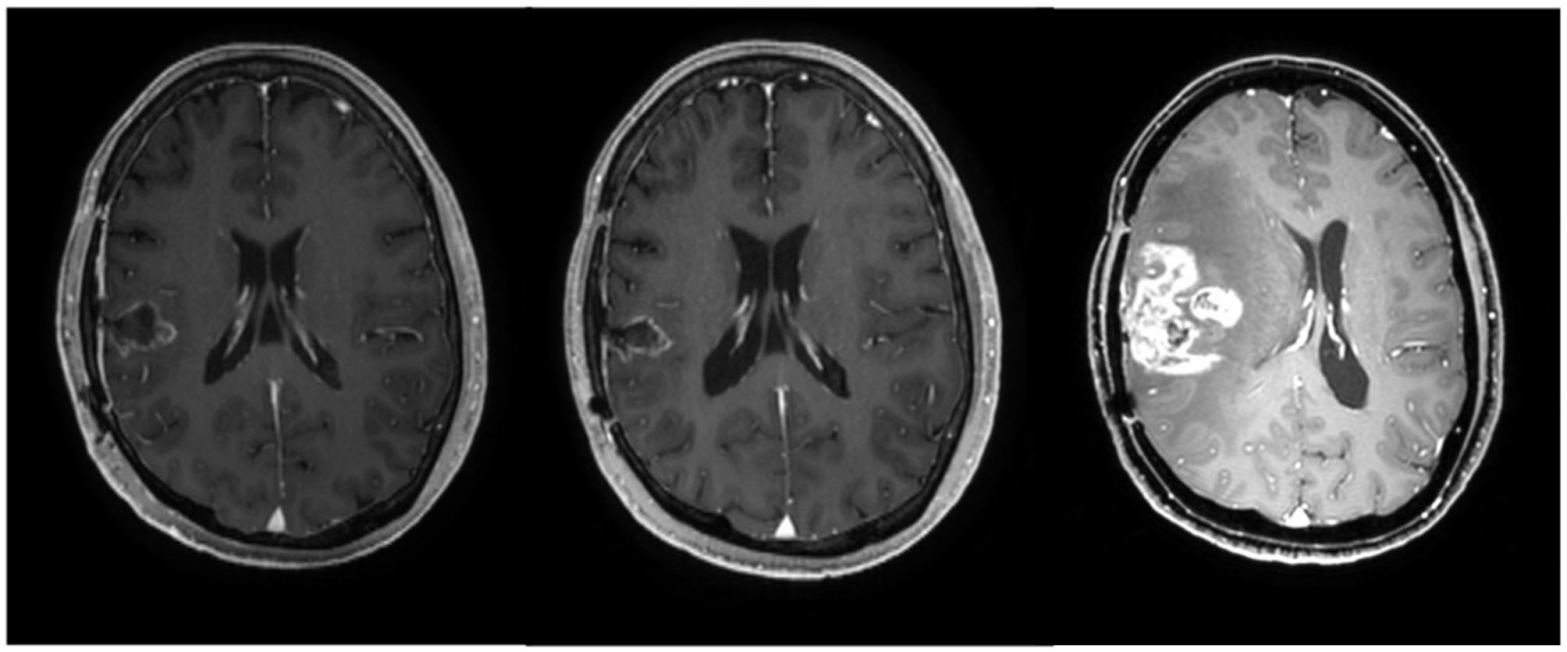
Axial MRI images of patient 6 at 3 months (before chemotherapy), 9 months (after the completion of chemotherapy), and 11 months after the initial diagnosis of glioblastoma, respectively.
